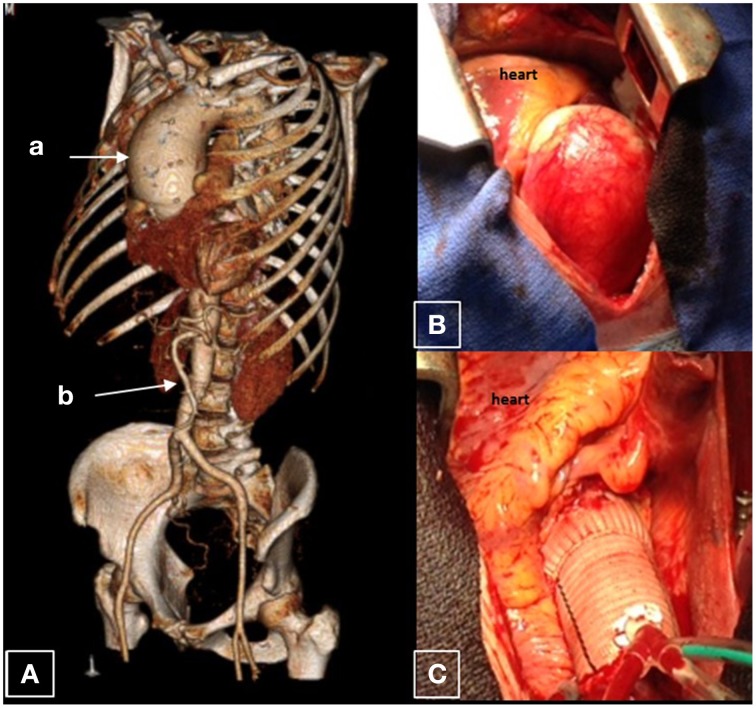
Ascending aortic aneurysm. This is an aorta of a 37 year old male who was found to have a 7.7 cm saccular ascending aortic aneurysm associated with a leaking bicuspid aortic valve. The remainder of the aorta is normal caliber. (A) CT reconstruction of the whole aorta with ascending aortic aneurysm (a), with a normal caliber abdominal aorta (b). (B) Surgical view of this aneurysm; (C) Dacron graft after surgical replacement.
